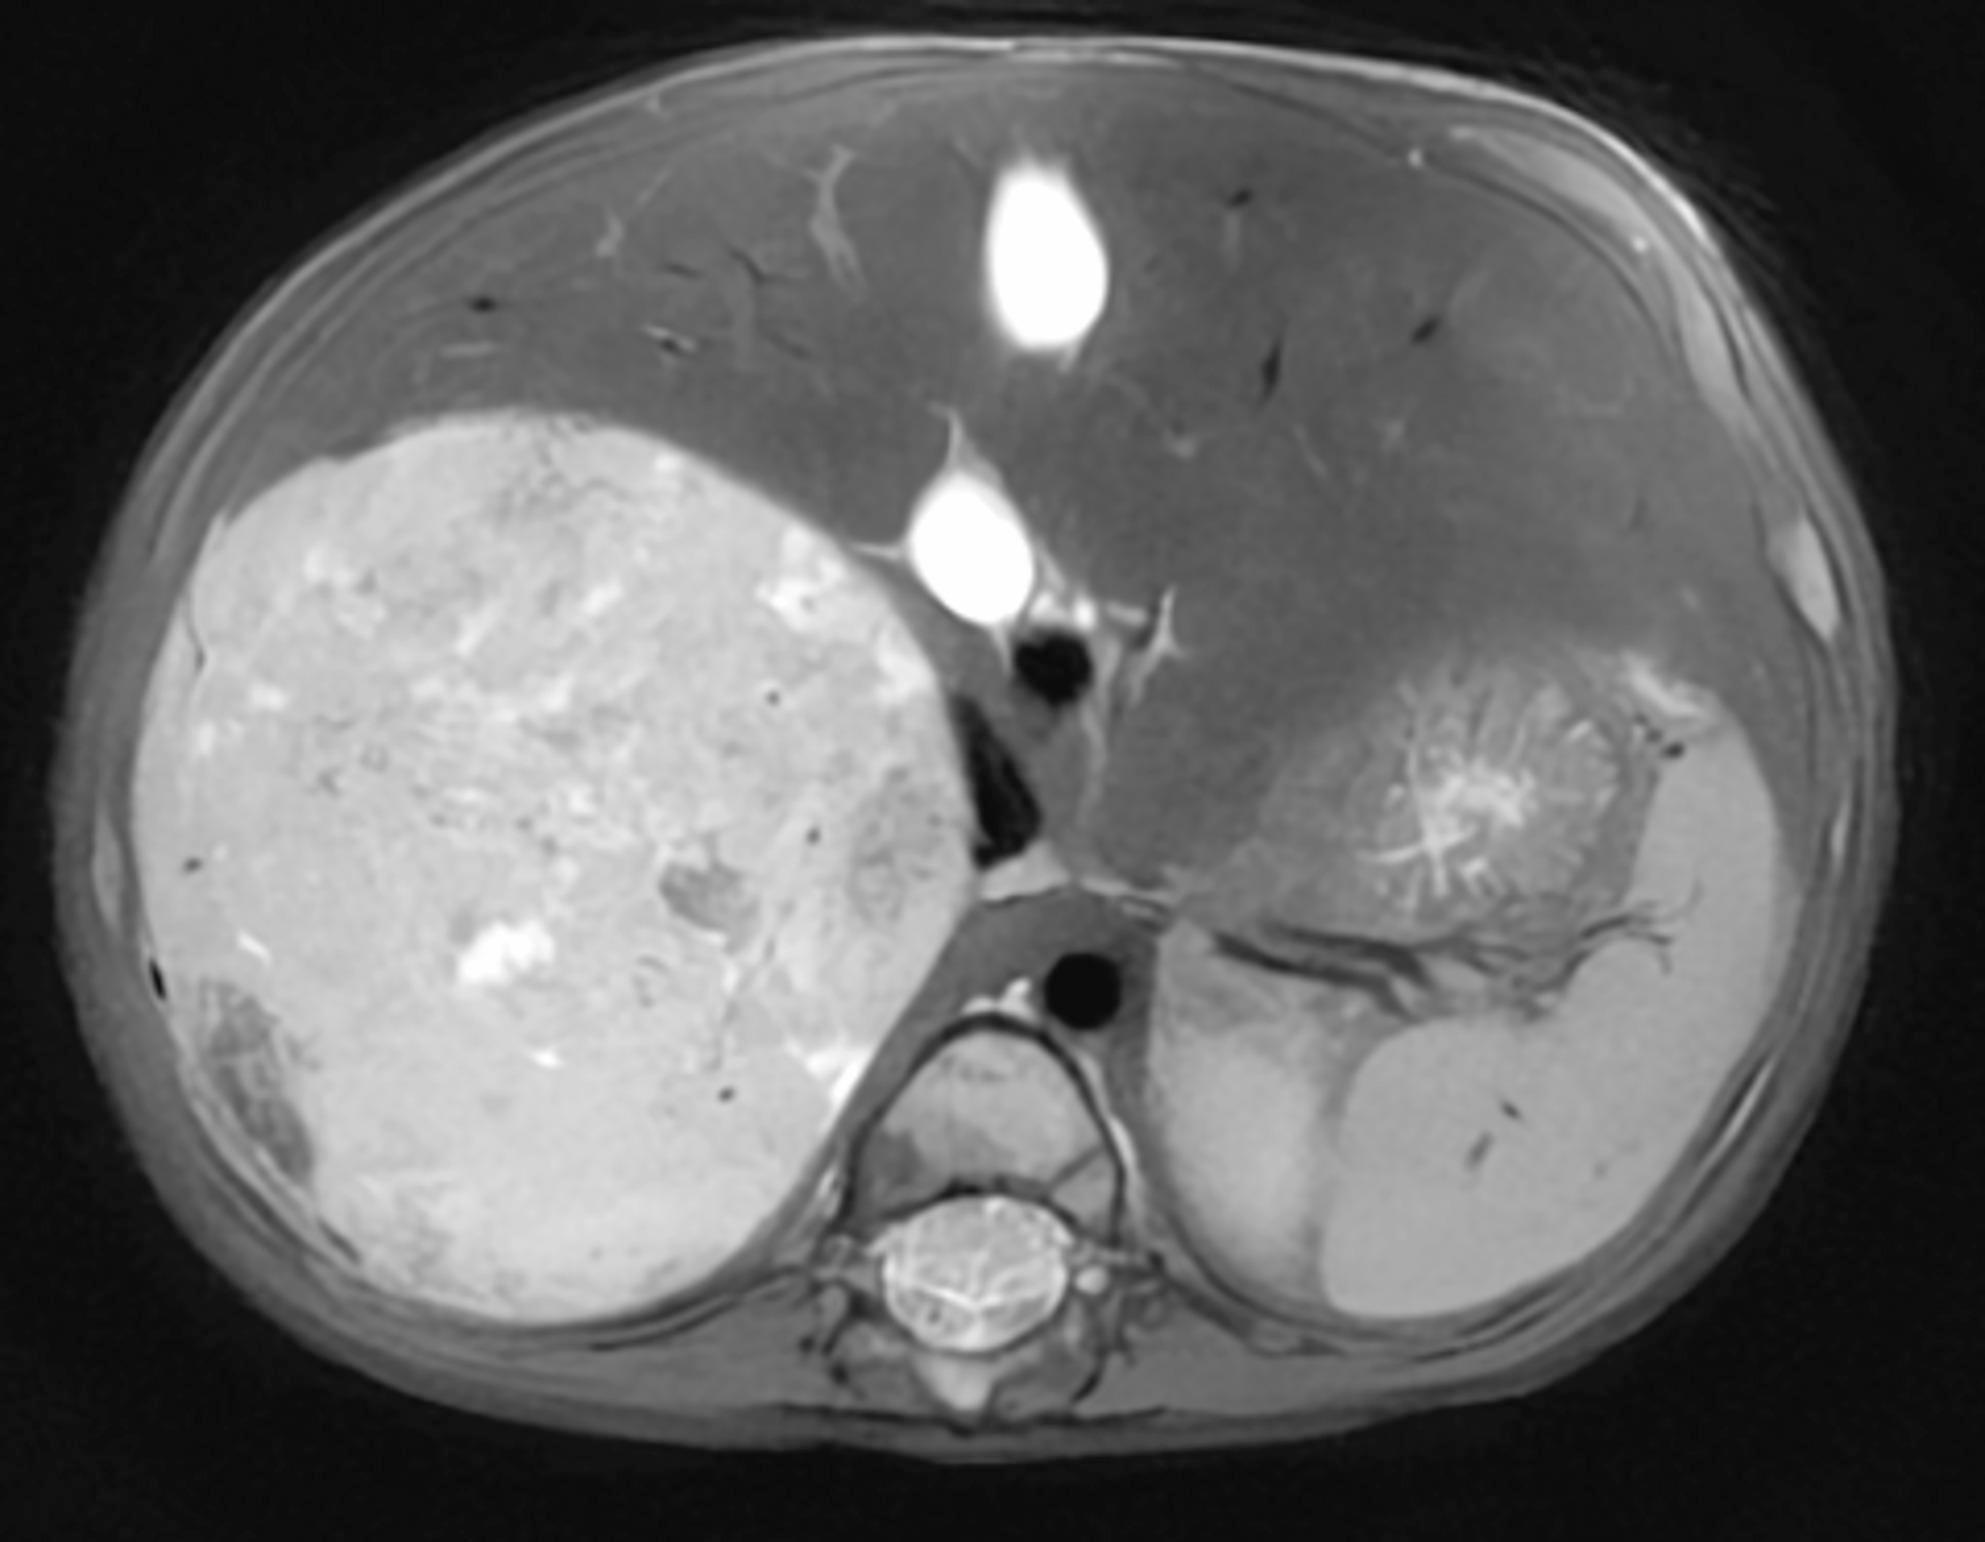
Ultrasound of the liver and biliary system performed on the day of referral, demonstrating an 8 × 7.5 × 8 cm heterogeneous, rounded mass in the right hepatic lobe, suspicious for hepatoblastoma or NBL
